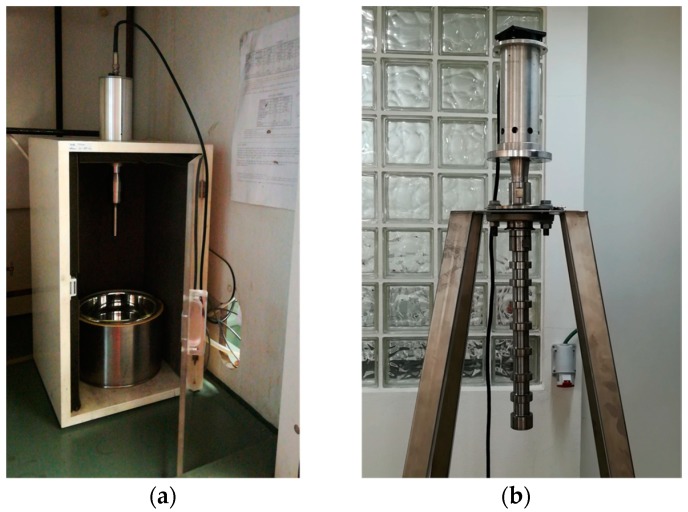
Probes used for the ultrasound-assisted extraction at: (a) lab scale; (b) pilot scale.
